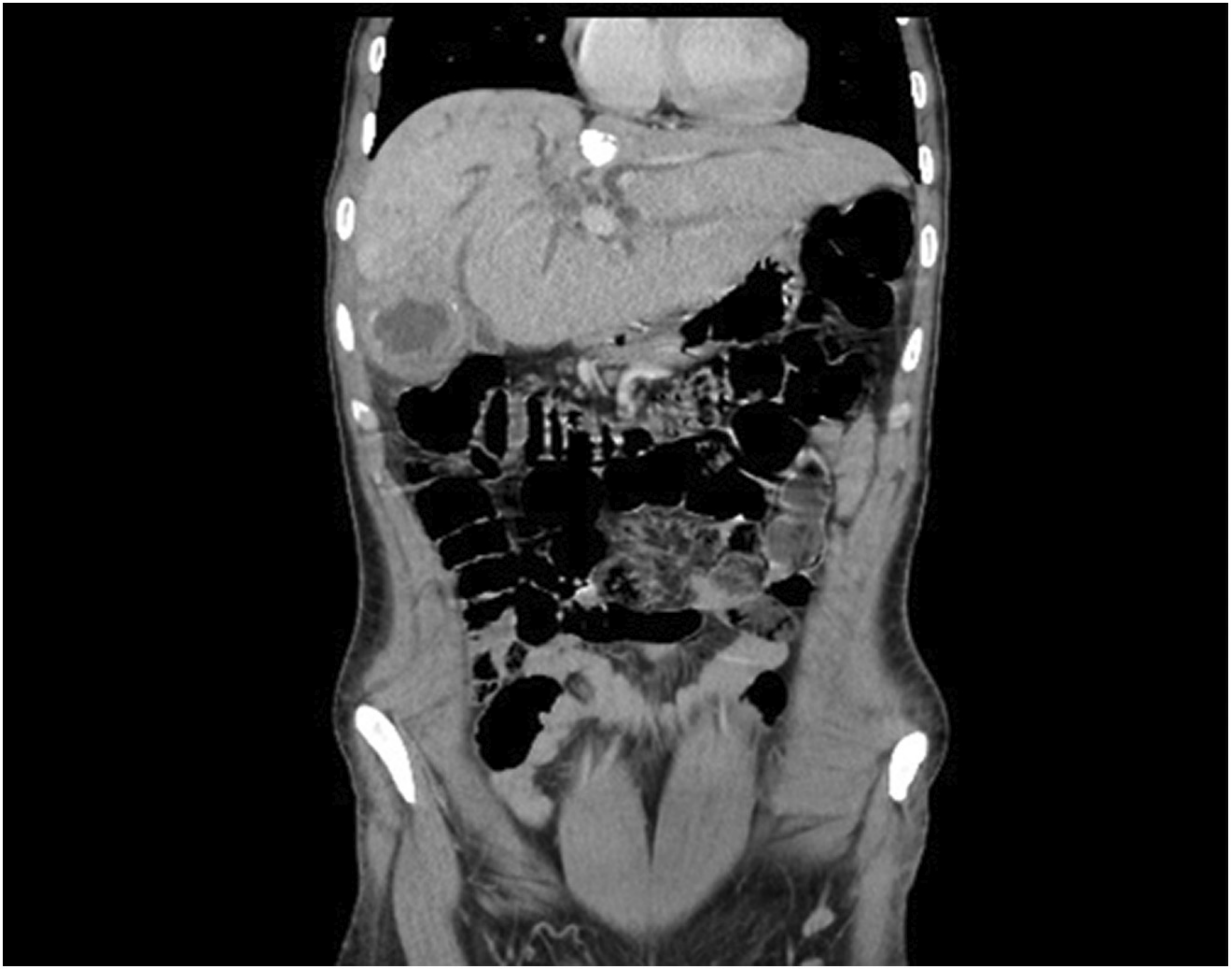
Axial abdominal CT SCAN showing a calcified lesion measuring about 2.5 × 1.3 cm in segment 4a.
